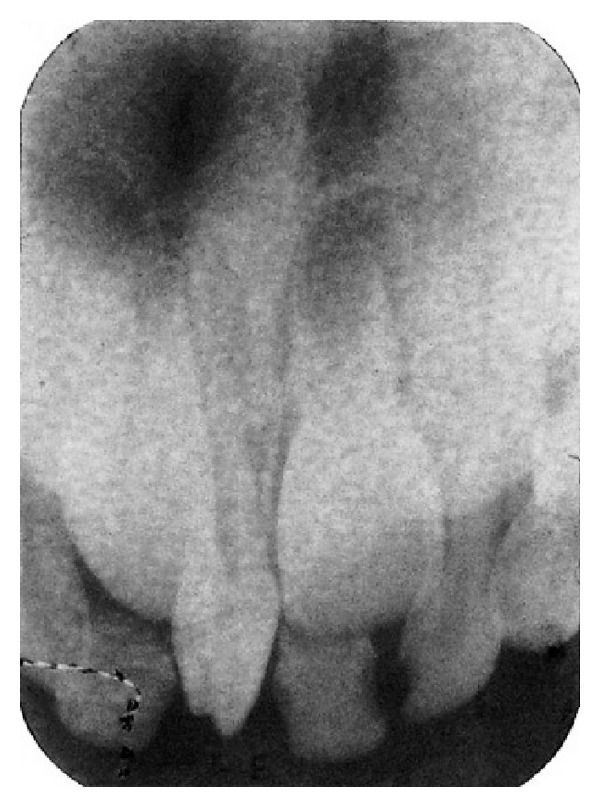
IOPA radiograph showing multilobulated mesiodens between maxillary primary central incisors.
